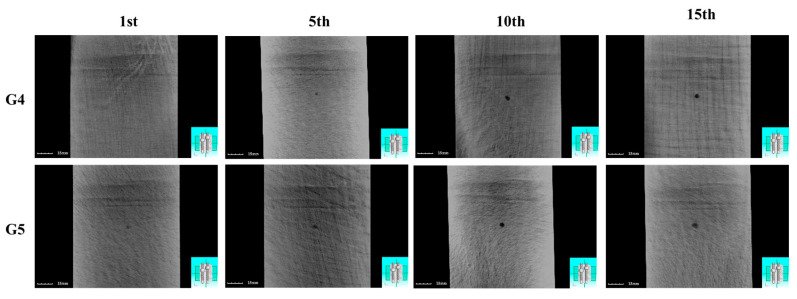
X-ray CT scans of CS and SS laminates under 5 J repeated impacts (1st, 5th, 10th, 15th).
